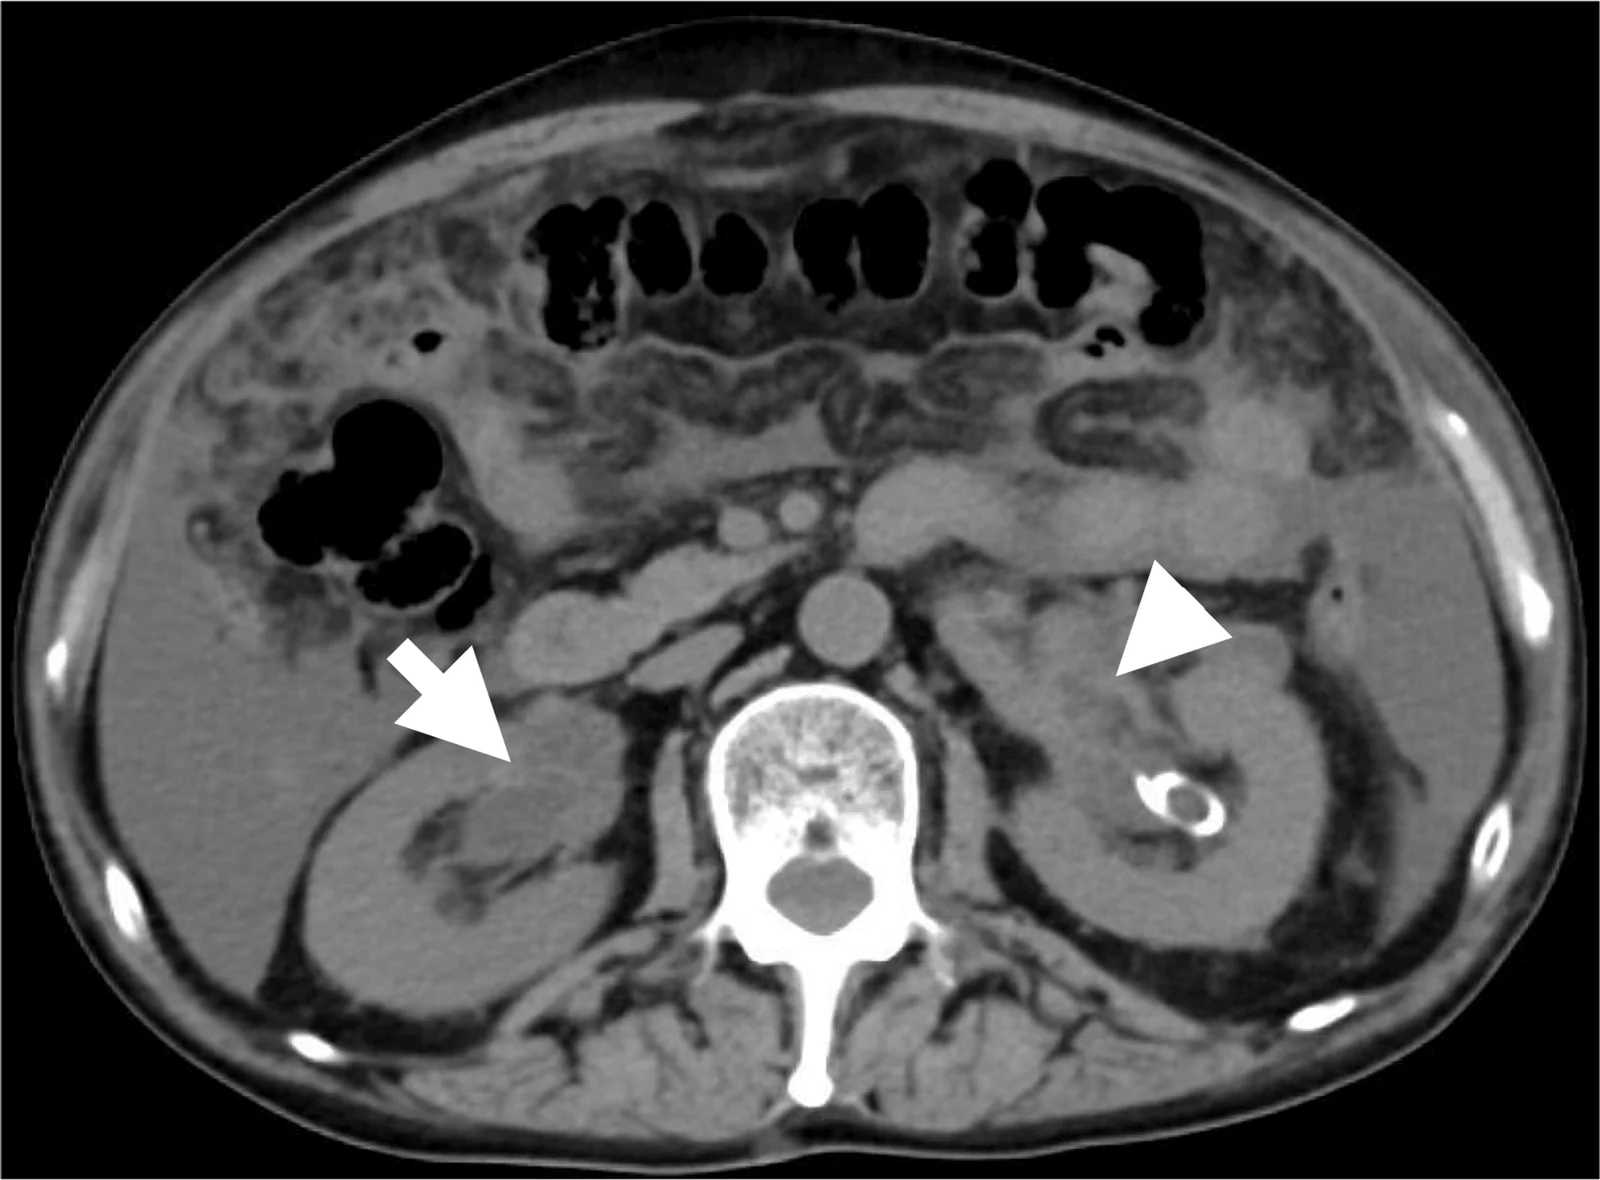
Bilateral renal metastases. Non-contrast CT showing bilateral perirenal lesions after left ureteral stent placement. The white arrow indicates the right lesion, and the white arrowhead indicates the left lesion.
